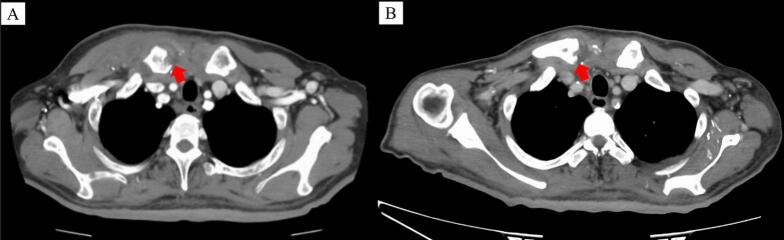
(A) Computed tomography shows erosions (a red arrow) of the right sternoclavicular joint. (B) Erosions (red arrows) remain at the last dose of vancomycin. (For interpretation of the references to colour in this figure legend, the reader is referred to the web version of this article.)
